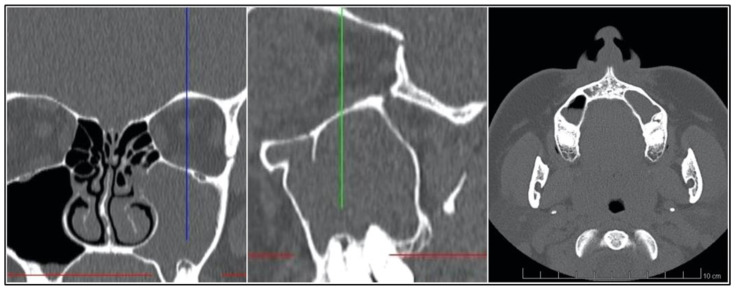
The example of CT scans of odontogenic maxillary sinusitis with a periapical lesion affecting mesio- and distobuccal root of first maxillary molar; from left to right: coronal, sagittal and axial view. Vertical and horizontal lines point the PAL.
